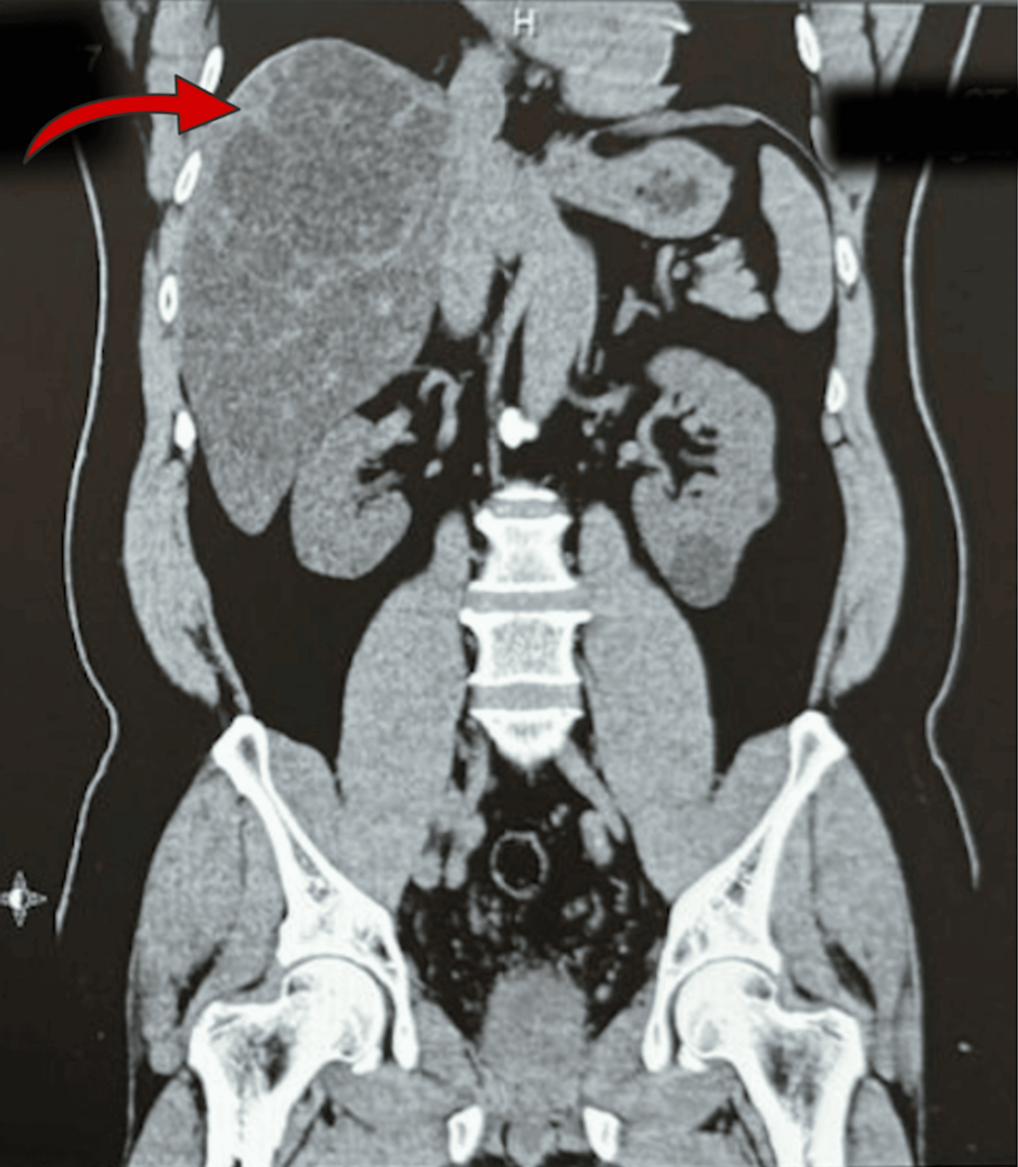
Computed tomography (CT) of the abdomen (coronal view) Demonstrating a large, well-defined multiloculated (hypodense) cystic lesion within the right hepatic lobe, with associated hepatic steatosis and posterior displacement of the inferior vena cava.
